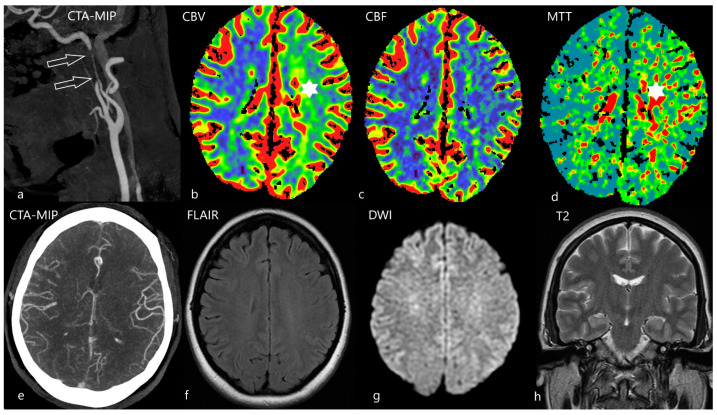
The effect of symmetric collaterals. 40-year-old female referred to emergency for unclear weakness of the right sided limbs and very discrete expressive aphasia that was mentioned by the patient herself since the previous evening. On the CT angiography (CTA) with maximum intensity projection (MIP), stenosis of the left internal carotid artery was found (arrows); dissection was suspected (a). CTP revealed apparent discrete signs of hyperperfusion on cerebral blood volume [CBV, (b), star], no abnormalities were apparent on cerebral blood flow [CBF, (c)], mean transit time (MTT) was slightly prolonged [(d), star]. Collaterals on CTA with MIP reconstruction were symmetric (e), on MRI scan (f–h) there were no signs of acute ischemia. She was treated by wall-stent insertion and the next day she was released on home care without any neurology symptoms. The source of dissection was not revealed.
